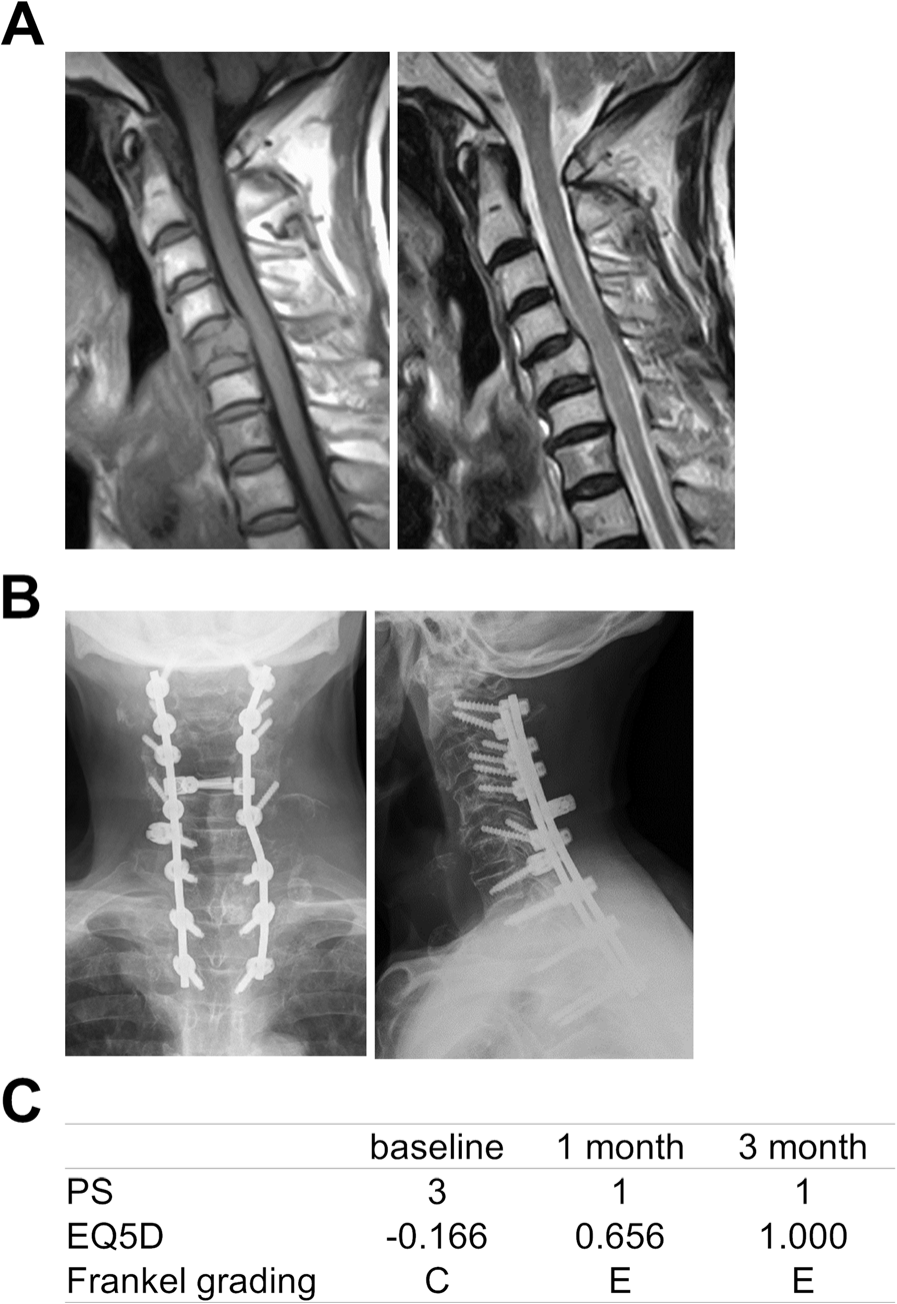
Images and clinical course of the 67-year-old man with metastatic thyroid cancer to the C5 vertebra. A Preoperative MRI: sagittal T1-weighed enhanced image at the left side and sagittal T2-weighed enhanced image at the right side. B Postoperative radiographs: posteroanterior image at the left side and lateral image at the right side. C PS, EQ5D, and Frankel grading preoperatively and at 1 and 3 months postoperatively. MRI, magnetic resonance imaging; PS, performance status; EQ5D, EuroQol 5-Dimension
